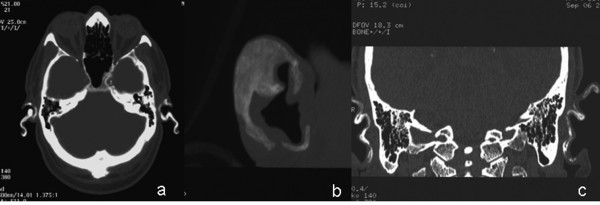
CT images. a. Axial CT images demonstrate ossification along both auricles. b. 3D images: complete ossification of both auricles. c. Tiny radiolucent areas within bony opacities are better seen in coronal reformatted images, indicating true bone formation.
